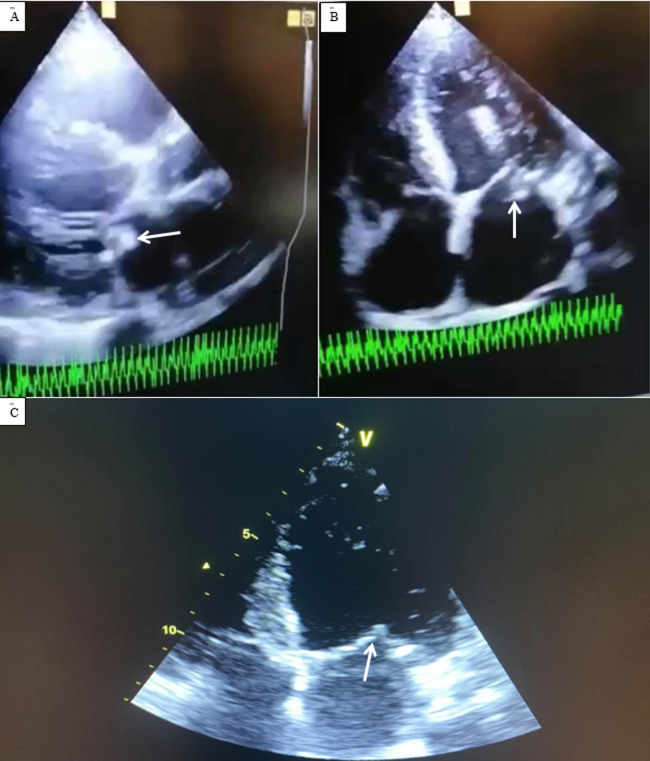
transthoracic echocardiogram: A) showing vegetation at the expense of the posterior mitral valve (white arrow); B) the four-cavity cut confirming vegetation at the level of the posterior mitral valve (white arrow); C) shows the disappearance of vegetation
